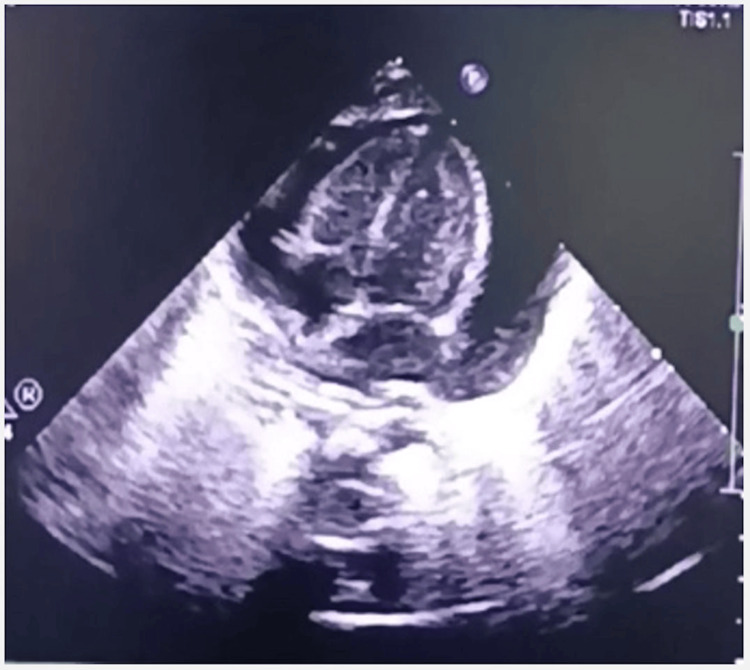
Echocardiogram and chest radiograph of Case 4 Echocardiogram showing PCE with CT PCE: Pericardial effusion; CT: Cardiac tamponade
